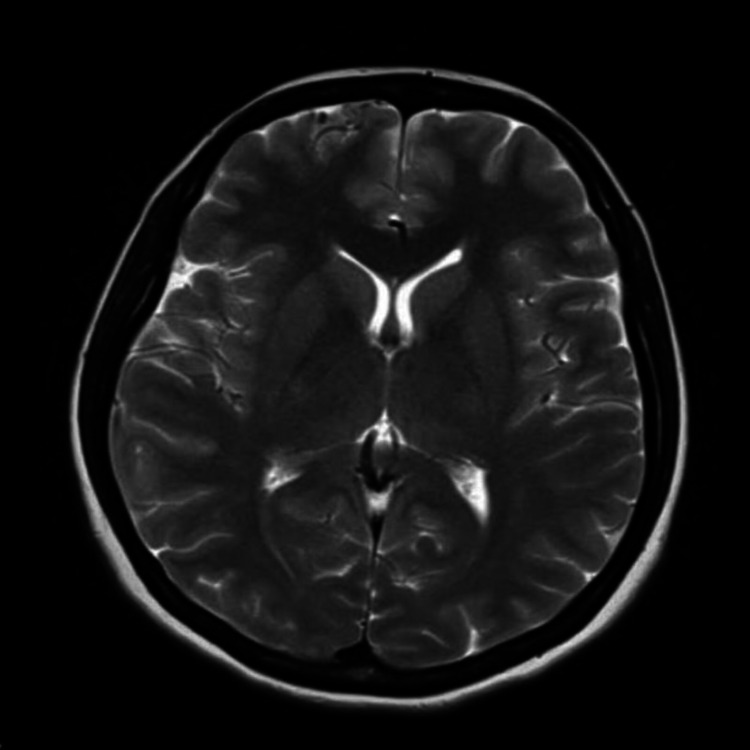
MRI showing right frontal arteriovenous malformation (case 4) T2-weighted axial section of MRI, showing evidence of a small bunch of vessels with a prominent dilated vein at the right frontal lobe anteriorly. Appearances are suggestive of small pial arteriovenous malformation.
